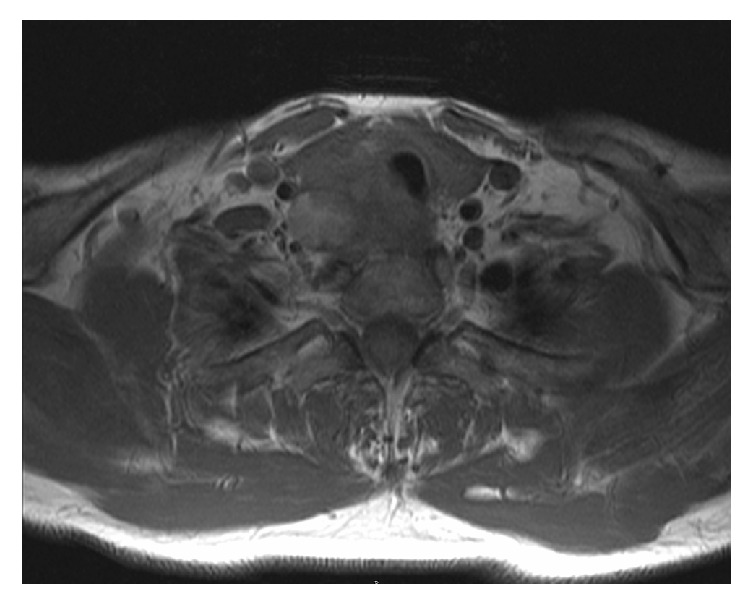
T1 MRI of neck showing invasion of trachea.
